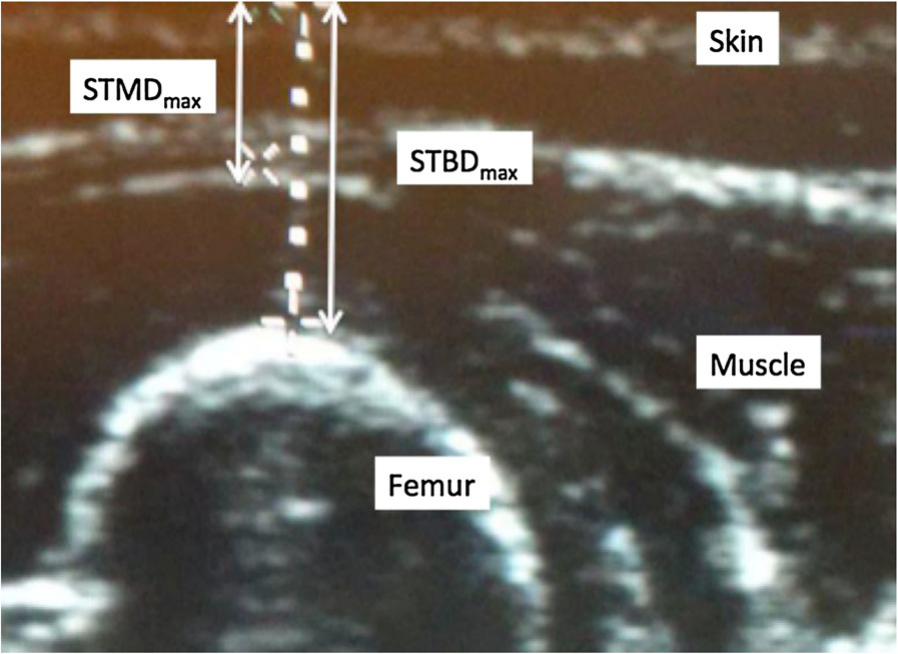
Ultrasound image of anterolateral thigh. STMD_max_: skin-to-muscle depth with maximal pressure; STBD_max_: skin-to-bone depth with maximal pressure.
